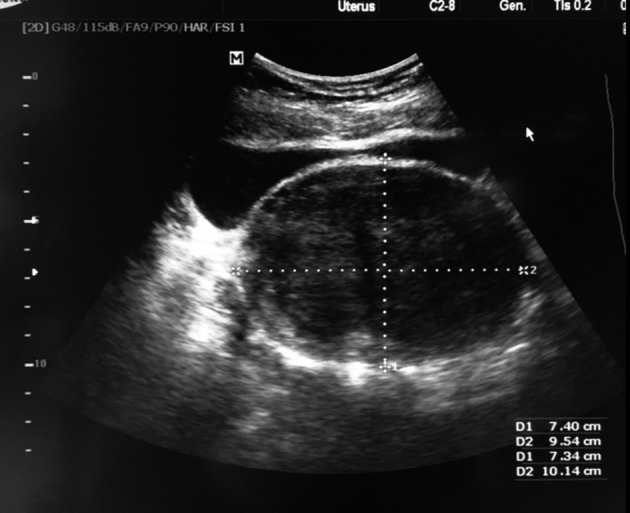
Ovaries were normal, but the uterus couldn’t be clearly identified. Laboratory work-up including full endocrine assessment and tumour markers was normal. Mayer-Rokitanski-Küster-Hauser (MRKH) syndrome was then suspected. An abdominal ultrasound showed both normally located kidneys. A pelvic MRI was suggested in order to prove the diagnosis and characterize the pelvic mass, but the lady refused it because of financial limitation. She asked to skip to a definitive diagnostic procedure. She was scheduled for a diagnostic laparoscopy as being part of primary amenorrhea work up and for the pelvic mass assessment. Diagnostic laparoscopy showed normal ovaries and fallopian tubes, the total absence of the uterus (Fig. 2) and a solid pelvic mass arising from the right-sided intra-peritoneal part of the round ligament (Fig. 3).
